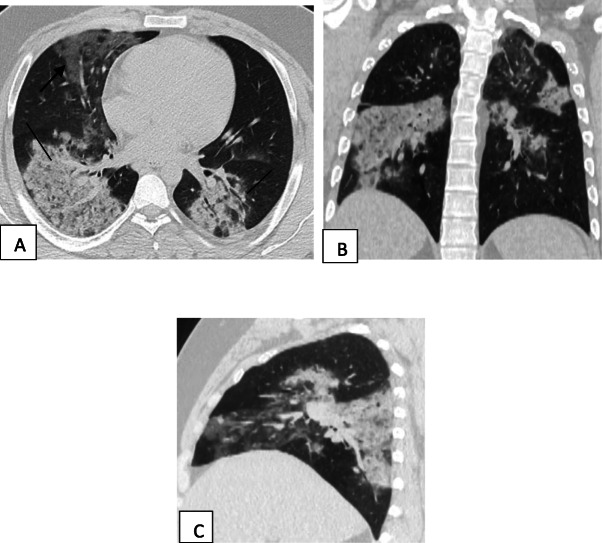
Unenhanced chest CT of a 21-year-old male patient with COVID-19. a An axial lung window image shows bilateral subpleural and broncho-vascular areas of ground glassing opacity [single head arrow] and crazy paving and consolidation [line]. b Coronal and c sagittal reformatted images. CT score is 18
